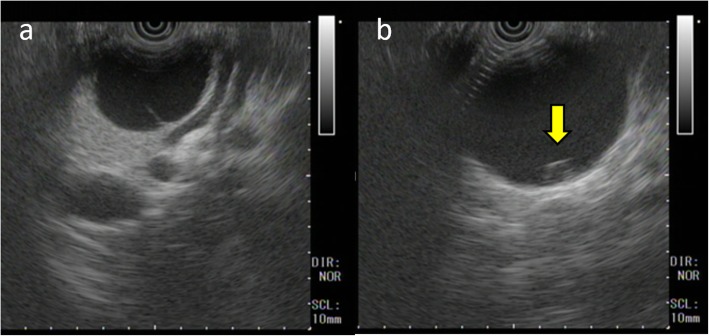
Endoscopic ultrasound revealed a multilocular cystic tumor (a) with nodular lesion (b, arrow).
